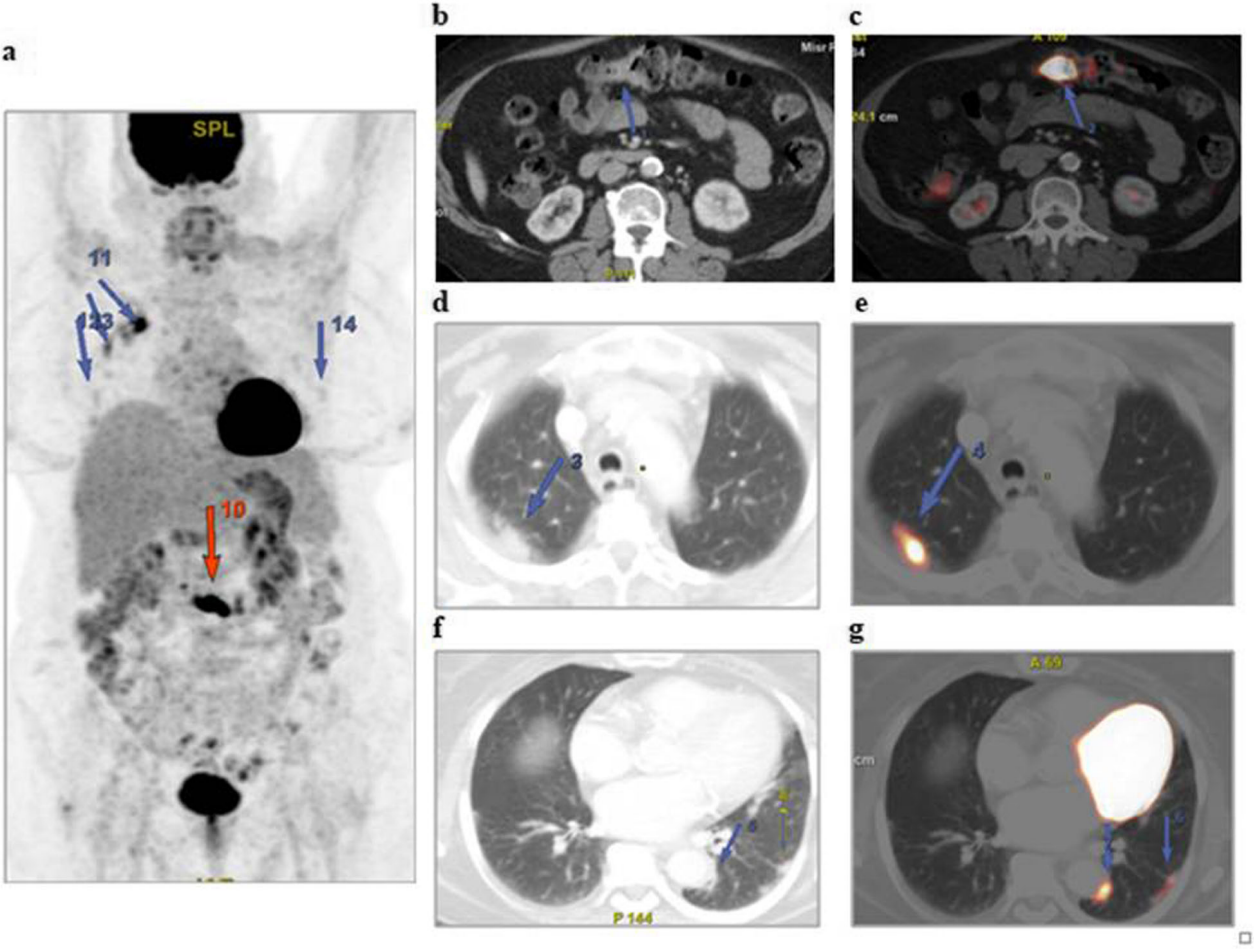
A 70-year-old female patient, presented with recently diagnosed cancer colon (adenocarcinoma grade II) and referred for initial PET CT evaluation. The whole-body MIP image (a) shows FDG avid lesion at the mid transverse colon (red arrow) with other bilateral pulmonary active lesions of variable FDG uptake (blue arrows). Axial abdominal CT and corresponding fused PET/CT images (b, c) revealed a short segment of FDG avid irregular circumferential malignant soft tissue mural thickening at the mid transverse colon. Axial lung window CT images (d, f) revealed bilateral subpleural ground-glass opacities and consolidations that show variable FDG avidity on corresponding fused PET/CT images (e, g) achieving up to 6.9 SUVmax
